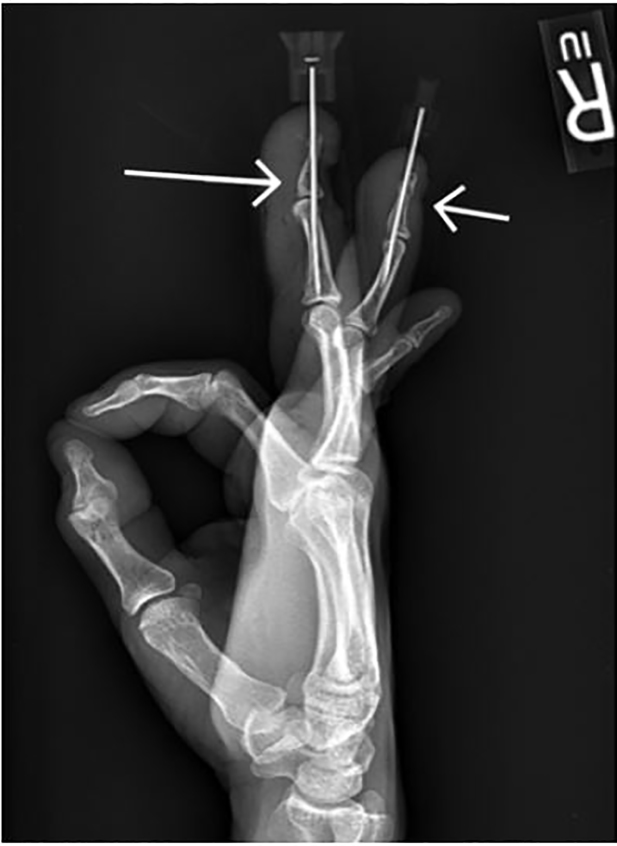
Post-repair radiograph of 3rd and 4th digits: 4th digit fracture pinned with 18-gauge needle (short arrow); 3rd digit mallet injury pinned in extension with IOVAD needle trocar (long arrow).
